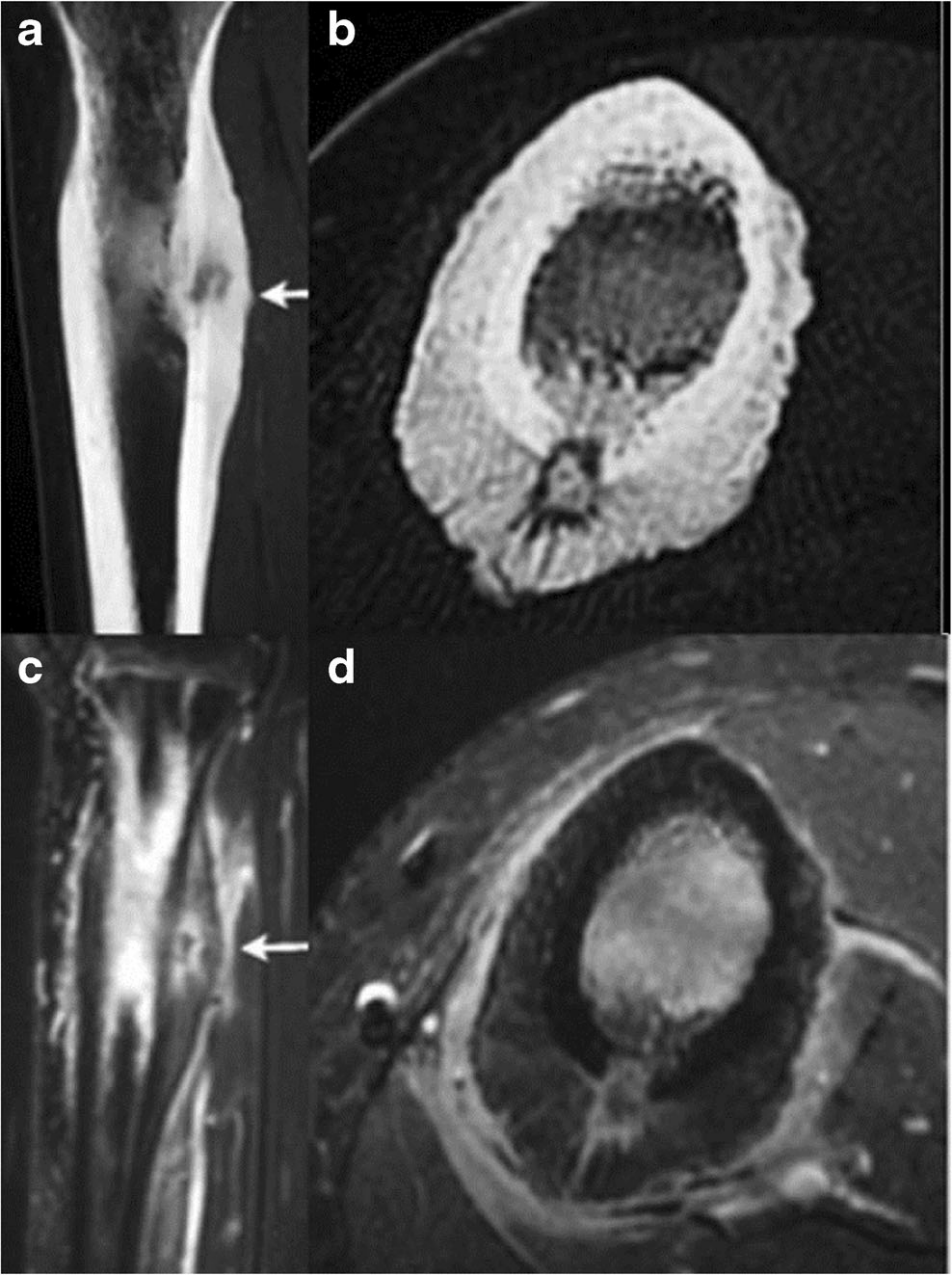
Sub-periosteal osteoid osteoma. A 15-year-old boy with sub-periosteal osteoid osteoma involving the right femur. Coronal a and axial b non-enhanced CT images show cortical thickening with reactive sclerosis (*arrow*) involving the postero-medial cortex of the femur, surrounding a low-attenuation nidus with central mineralization. Coronal STIR c and axial T1 post-contrast d images show avid contrast enhancement and edema within the bone marrow and soft tissue surrounding the nidus. The periphery of the nidus enhances more intensely than the central portion (*arrow*)On MR, there is typically dense fusiform thickening of the cortex, which has low signal on T1W, PDWI, T2WI, and fat-suppressed T2WI. Within the thickened cortex, there is a spheroid or ovoid zone (nidus) measuring less than 1.5 cm. The nidus can have low–intermediate signal on T1WI and PDWI, and low–intermediate or high signal on T2WI and FS T2WI. Calcifications in the nidus can be seen as low signal on T2WI. After gadolinium contrast administration, variable degrees of enhancement can be seen at the nidus [2].Fibrous cortical defect (FCD)FCDs are benign fibrohistiocytic lesions in the metaphyseal portions of long bones. The pathology is similar to non-ossifying fibromas (NOF), with differences primarily relating to size; fibrous cortical defects are small intracortical lesions, whereas the larger non-ossifying fibromas are located eccentrically in the medullary cavity. Up to 95 % of these lesions occur between the ages of 5 and 20 years [2].On radiographs, these present as lucent lesions with a sclerotic rim (Fig. 5). Cortical thinning or thickening, and bone expansion can be seen, more commonly with non-ossifying fibromas, given their larger size [2]. On fat-suppressed T2WI, a sclerotic rim is of low signal intensity is often seen. Variable degrees of gadolinium contrast enhancement are present.
